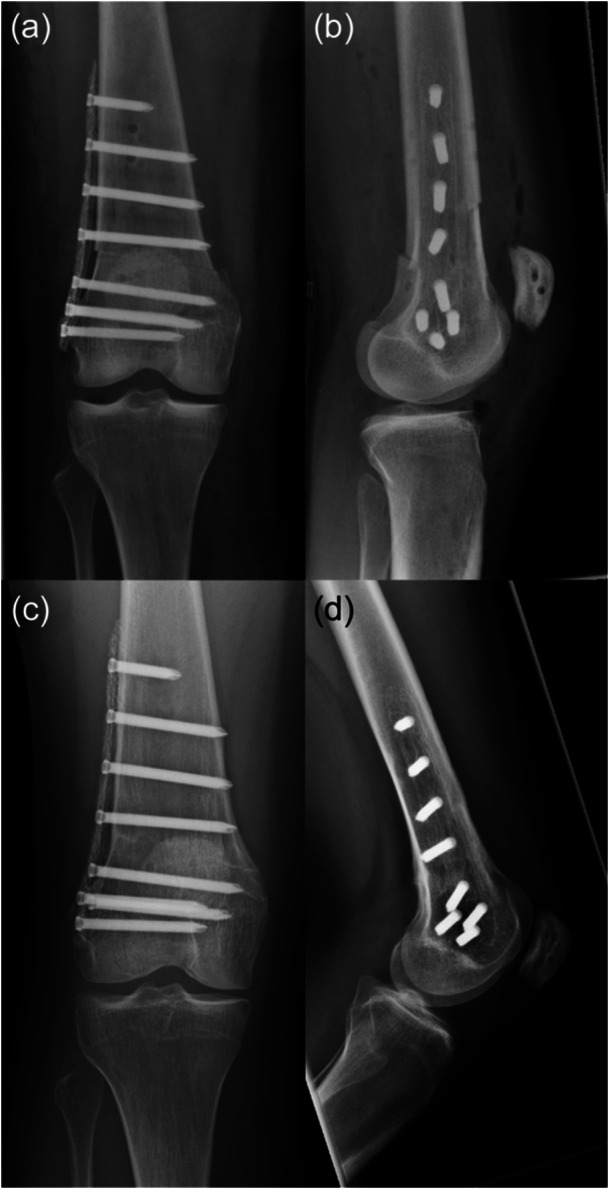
Clinical case of a 21‐year‐old female patient who underwent derotational distal femoral osteotomy with concomitant medial patellofemoral ligament reconstruction for the treatment of recurrent patellofemoral instability. (a, b) Plain radiographs 2 days post‐operatively; (c, d) plain radiographs 1‐year post‐operatively showing consolidated osteotomy.
